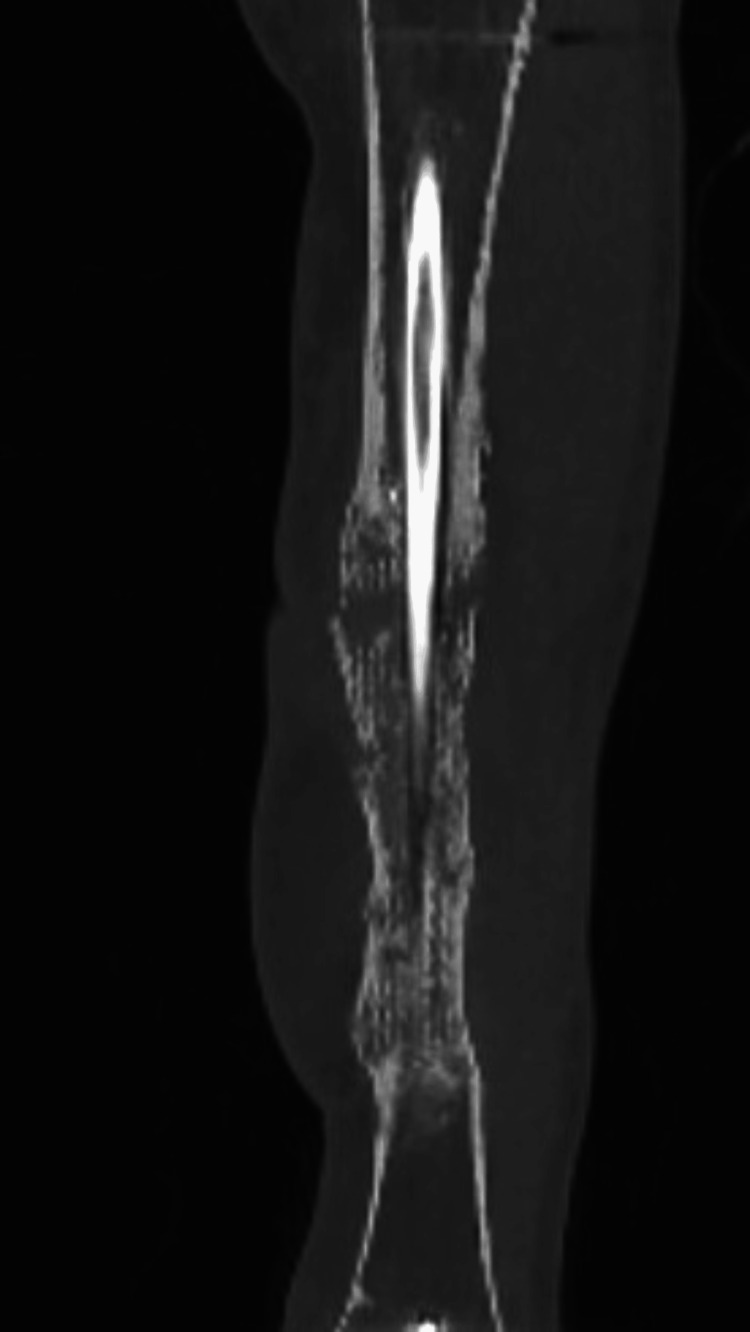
Case 1: Low-dose CT one and a half years after the second stage IMT. IMT: Induced membrane procedure
